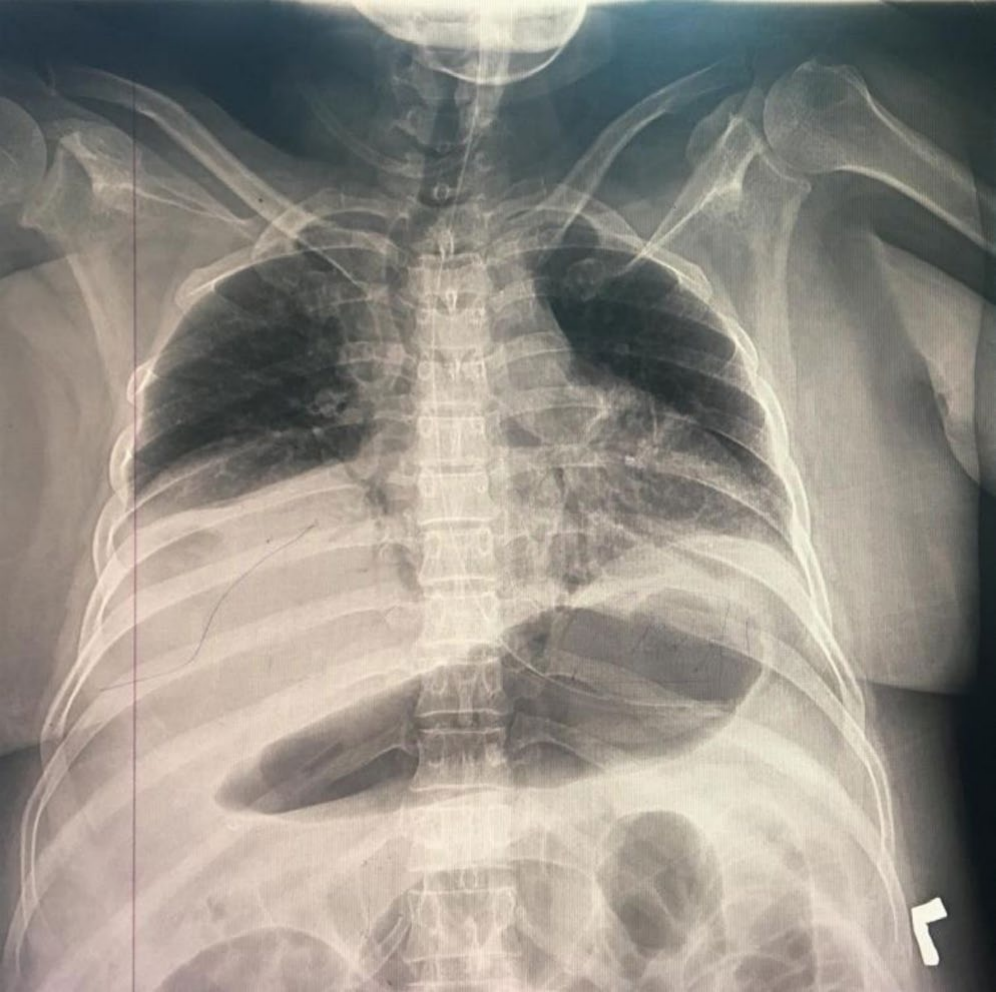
Anteroposterior (AP) supine chest radiograph of the patient on the third day of admission. The image demonstrates a significant left‐sided pleural effusion, a notable pleuropulmonary complication of severe acute pancreatitis. This finding is characterized by the opacification of the lower hemithorax and pronounced blunting of the left costophrenic angle, indicative of substantial fluid accumulation within the pleural space. The presence of such an effusion is consistent with the systemic inflammatory cascade precipitated by the underlying pancreatic pathology.
